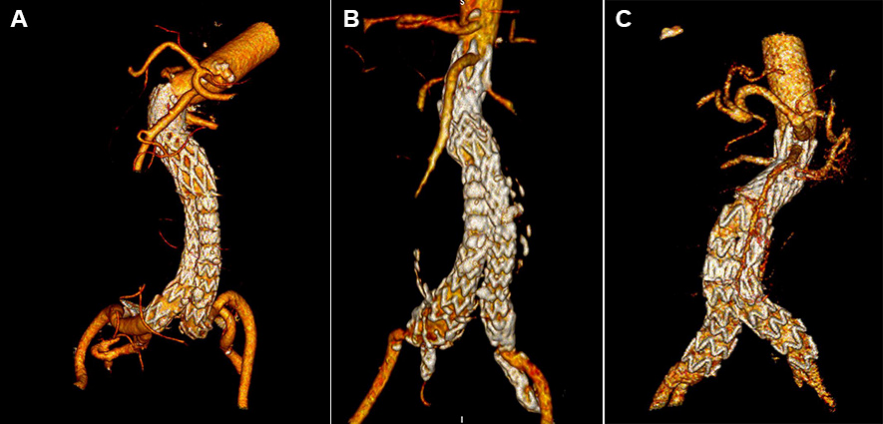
Postoperative 3D CT angiography reconstructions, 30 days after EVAR with the Neoneck technique. (A) 3D CT reconstruction of the first patient treated; (B) 3D CT reconstruction of the second patient treated; (C) 3D CT reconstruction of the third patient treated.
